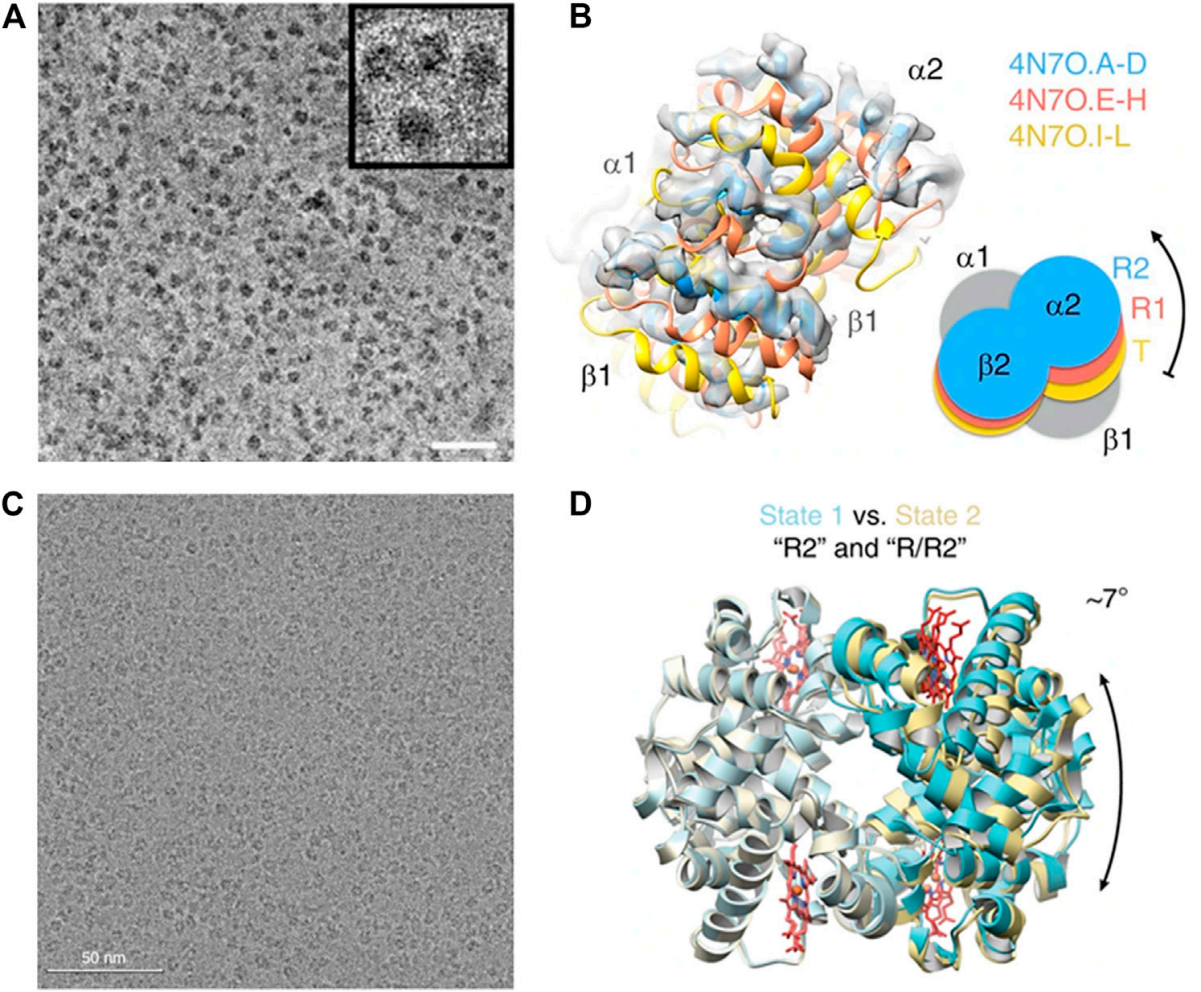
Single particle cryo-EM reveals co-exiting conformations of haemoglobin. (A) Visualization of haemoglobin with the aid of Volta phase plate (see the milestones in Figure 2B). (This figure is adopted from Figure 1A of Ref 41, an open access article distributed under the terms of the Creative Commons CC BY license. https://creativecommons.org/) (B) Modeling the cryo-EM map with known X-ray structures suggests the co-existing conformations. (This figure is adopted from Figure 1E of Ref 41, an open access article distributed under the terms of the Creative Commons CC BY license. https://creativecommons.org/). (C) Visualization of haemoglobin in thin ice without phase plate. This figure is adopted from Figure 1A of Ref 42. (D) Resolving the cryo-EM map with the usage of K-way 3D classification on RELION indicates the co-existing conformations. (This figure is adopted from Figure 1E of Ref 42, an open access article distributed under the terms of the Creative Commons CC BY license. https://creativecommons.org/).
